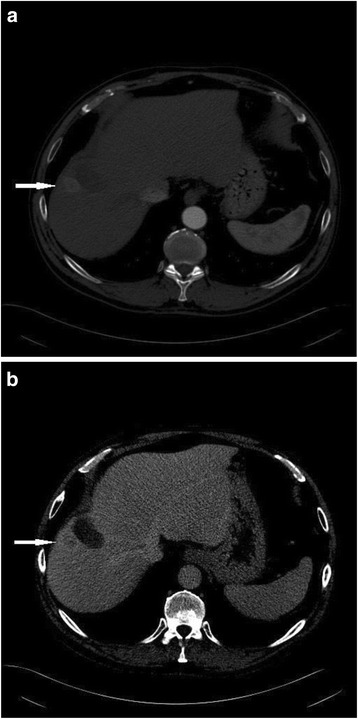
57 year old female with cirrhosis and HCC treated with RFA. CT in arterial (a) and venous (b) phases shows enhancement and washout of a nodule adjacent to an RFA ablation zone
